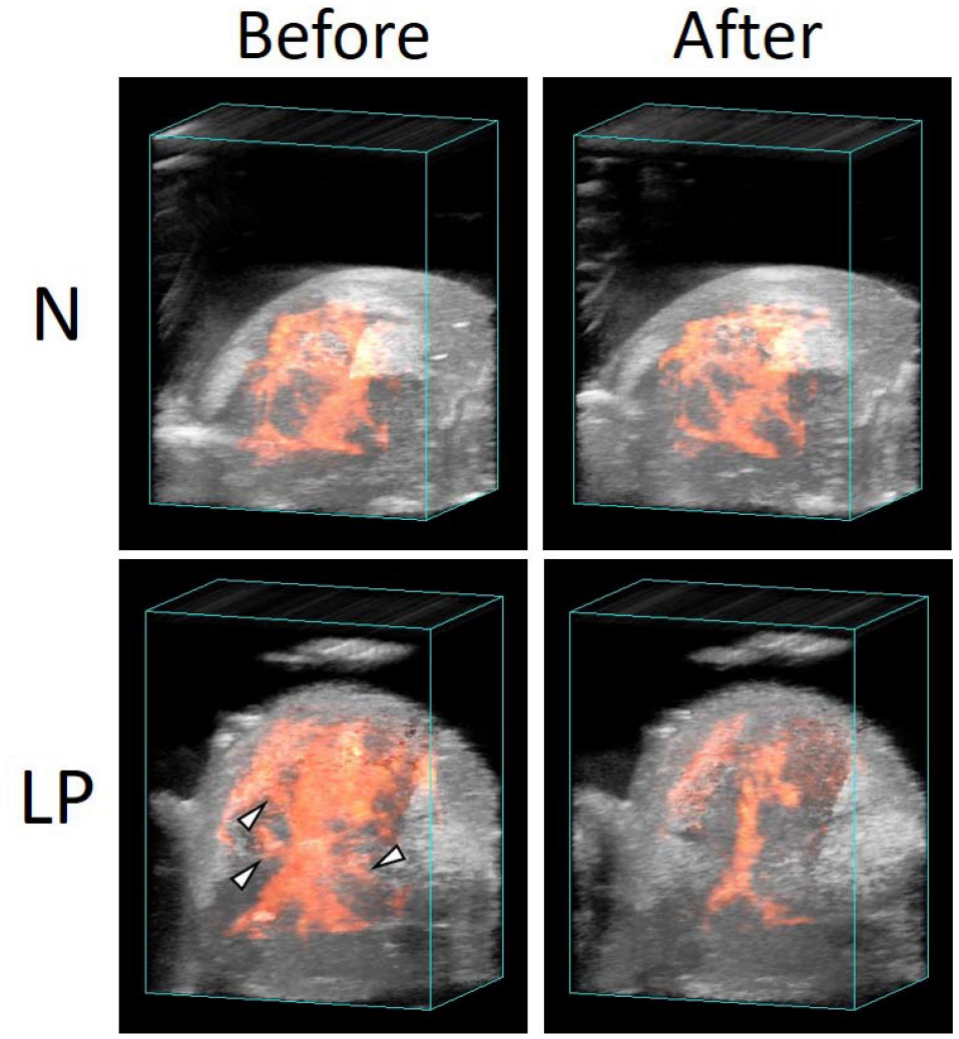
Vascular structures in normal (N) and low protein (LP) fetal brains before and after the ischemia/reperfusion treatments, reconstructed by 3D power Doppler imaging.
